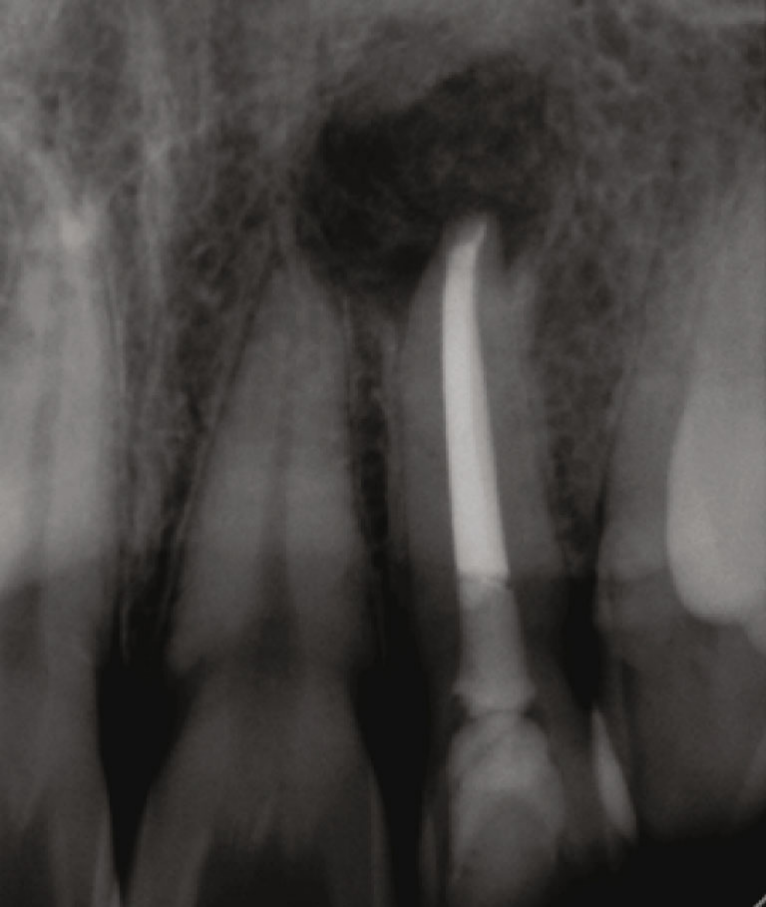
Posttreatment radiograph after 6 months.
